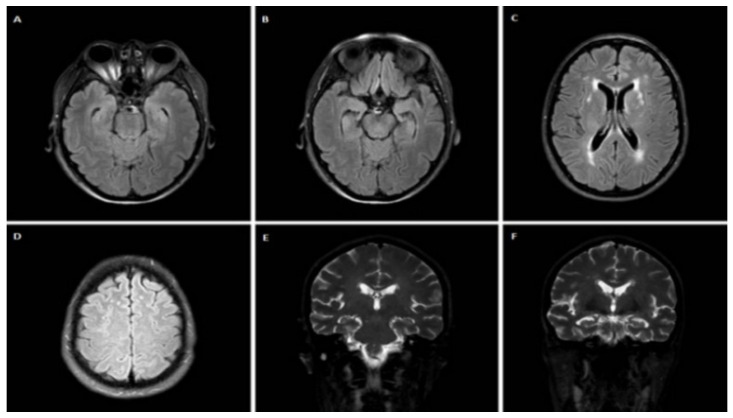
Brain MRI of case 4. Axial FLAIR images (A–D) and coronal T2 images (E,F) reveal hyperintense lesions attributed to chronic small vessel ischemia (Fazekas II) and some degree of cortical atrophy in temporal lobes.
